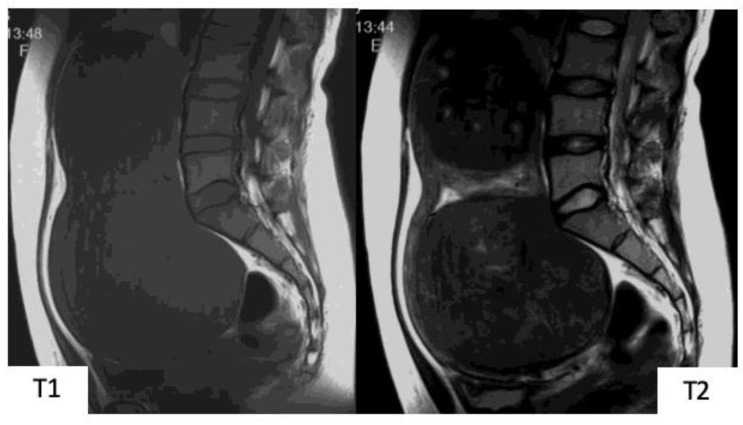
Typical images of uterine myoma in magnetic resonance images. In T1-weighted images, signals equivalent to or relatively lower than that of the normal myometrium can be observed. In T2-weighted images, signals that are clearly lower than those of the normal myometrium, with resection of almost all spherical masses with clearly defined borders, can be observed.
